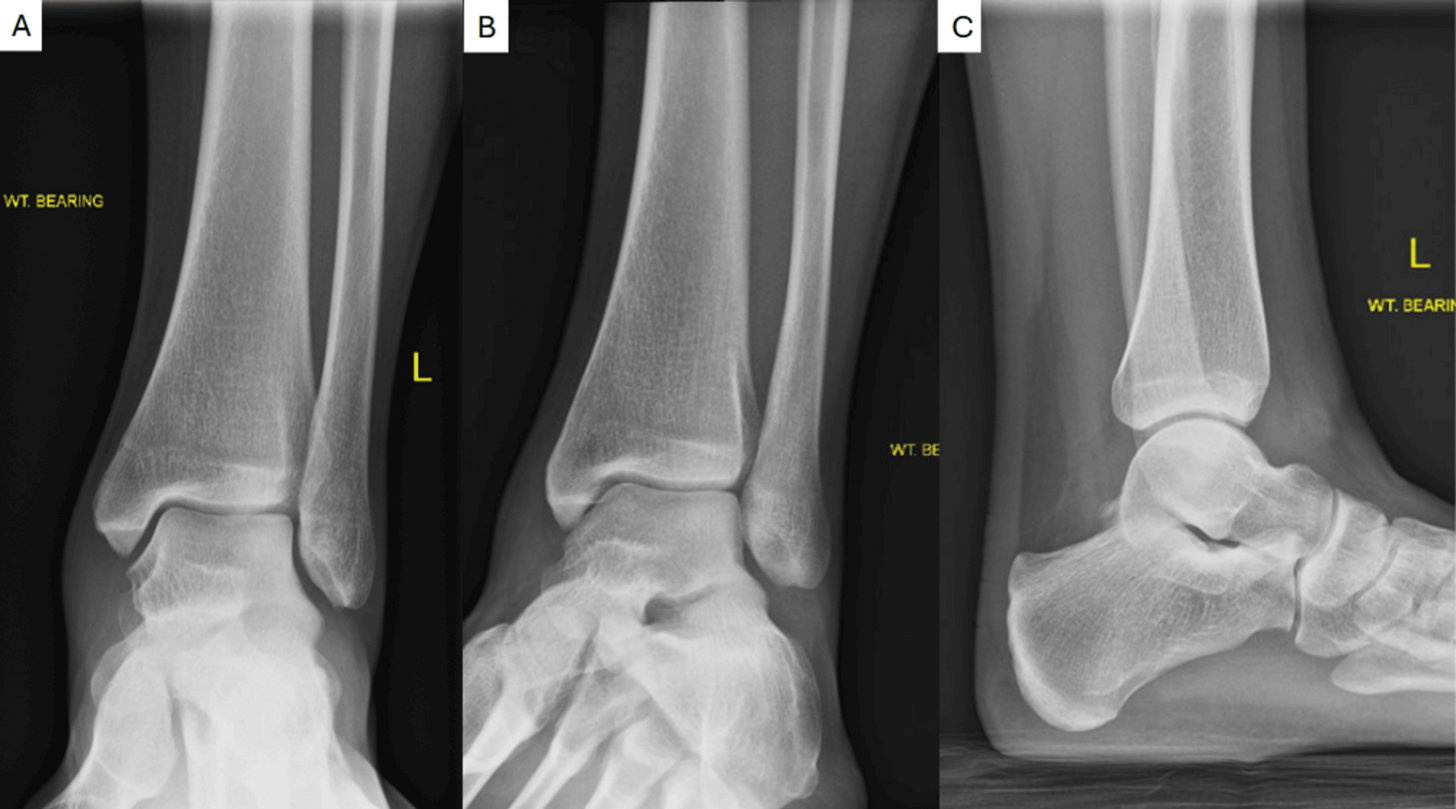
Anteroposterior (A), oblique (B), and lateral (C) weightbearing radiographs of the left ankle.
